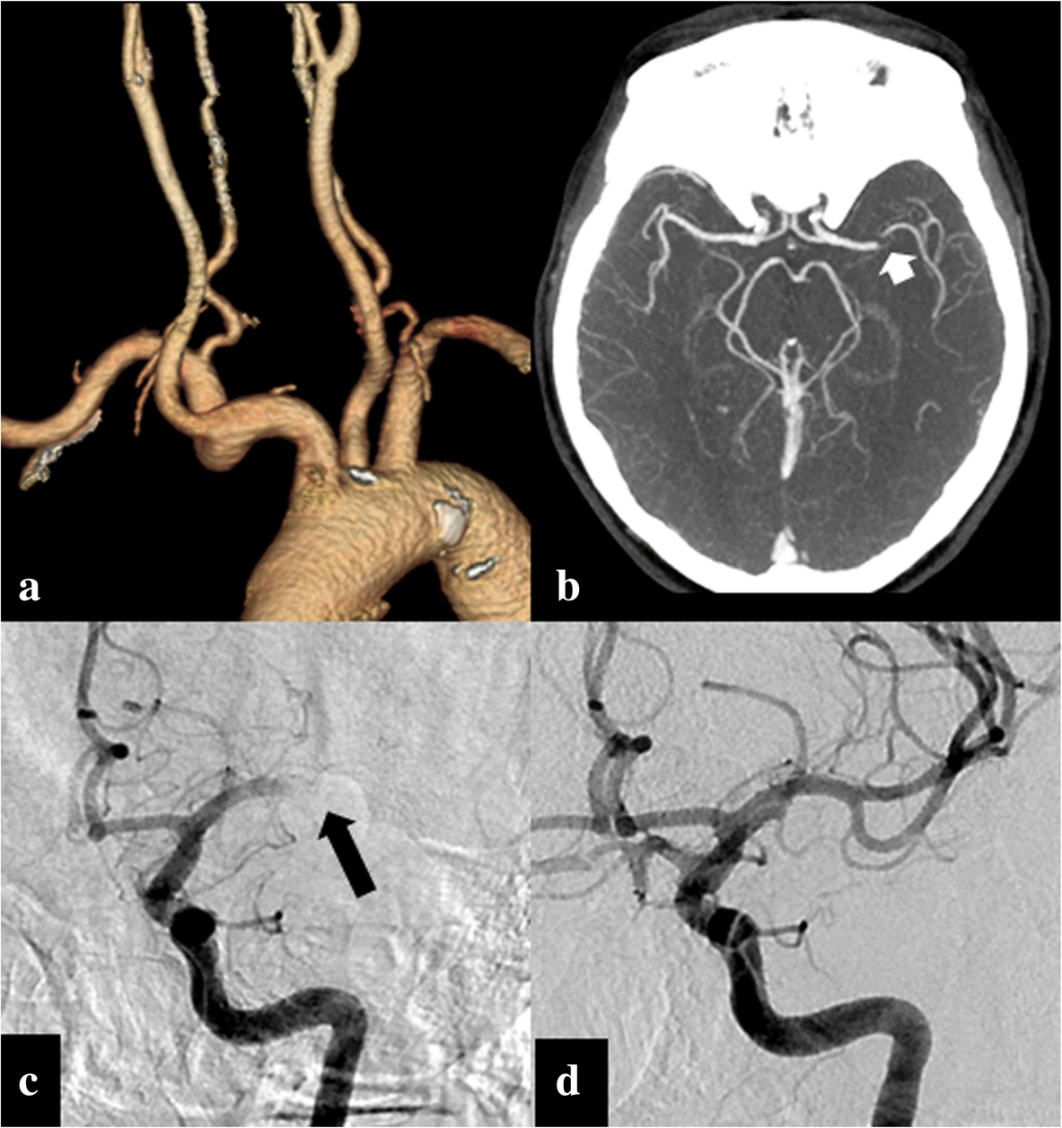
This 78-year old woman with atrial fibrillation presented with sudden onset of right-sided hemiplegia and aphasia. CT-angiography (CTA) with 3D volume rendering shows normal patency with moderate tortuosity of the cervical carotid arteries (a). An axial maximum intensity projection (MIP) reformation of the CTA demonstrates an occlusion in the M1 segment of the left MCA (b, white arrow). The patient was referred immediately for EVT. Catheter digital subtraction angiography, in anteroposterior (AP) projection, confirmed the left MCA M1 segment occlusion (c, black arrow). Subsequently, a successful endovascular removal of the thrombus was performed (d, post EVT AP projection angiography). At clinical follow-up, a complete resolution of the neurological symptoms occurred soon after EVT
